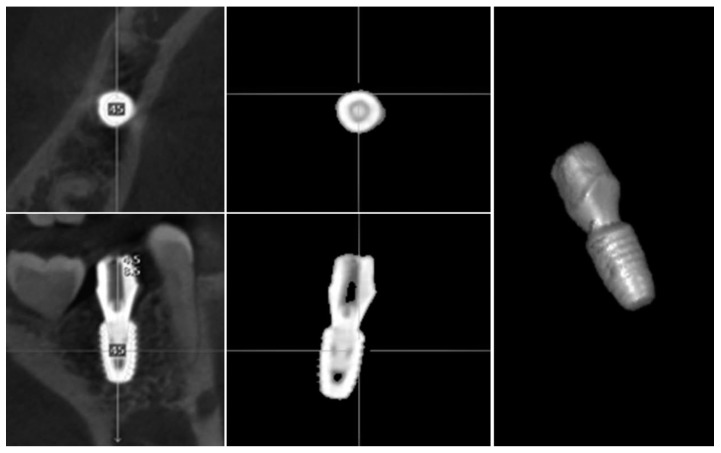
A portion of implant on the post-operative computed topography (CT) is separated. 3D modeling analysis confirms the actual position by directly reproducing the shape of the implant.
